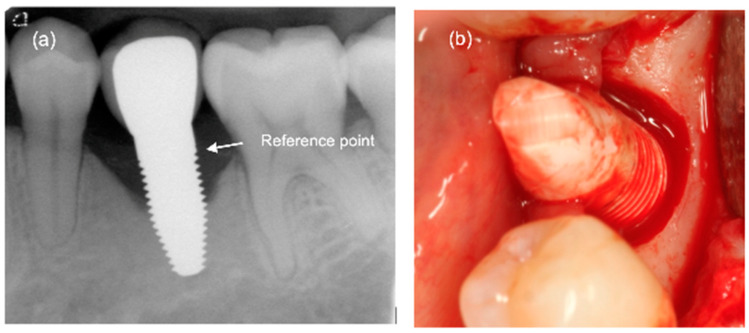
(a) Exemplary radiograph at the 5-year follow-up depicting a single-tooth implant with a distinct vertical defect at the mesial and distal aspect of the implant. The lower corner of the straight cylindrical implant part was used as reference point for bone level calculations. (b) Clinical situation of the same implant prior to removal.
